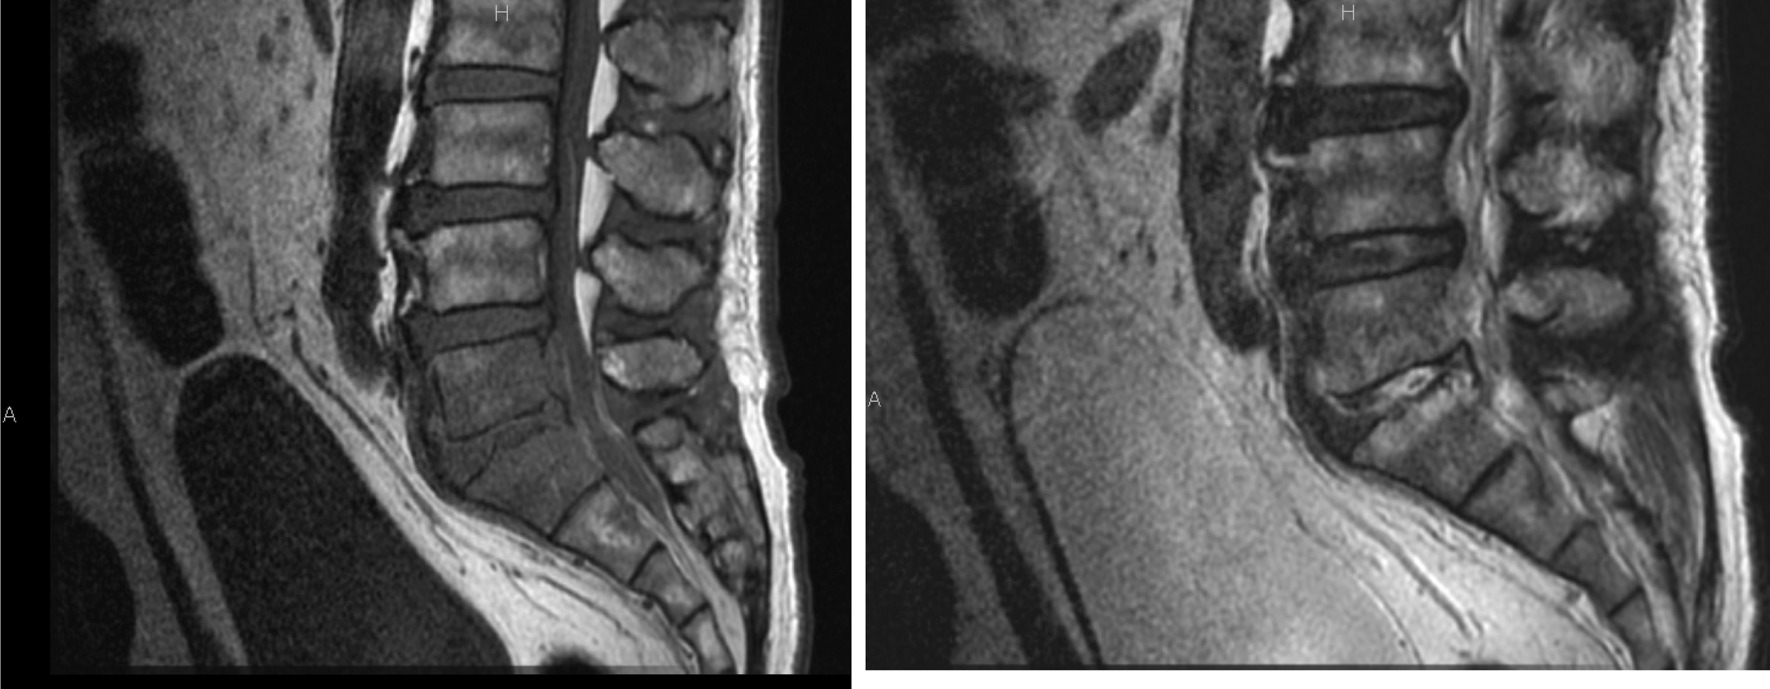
T1-weighted MRI (Left) and T2-weighted MRI (Right) images of suspected osteomyelitis at L5 and S1. T1-weighted MRI (Left) and T2-weighted MRI (Right) showed that intensity of L5 and S1 vertebrae were altered and vertebral osteomyelitis was suspected
